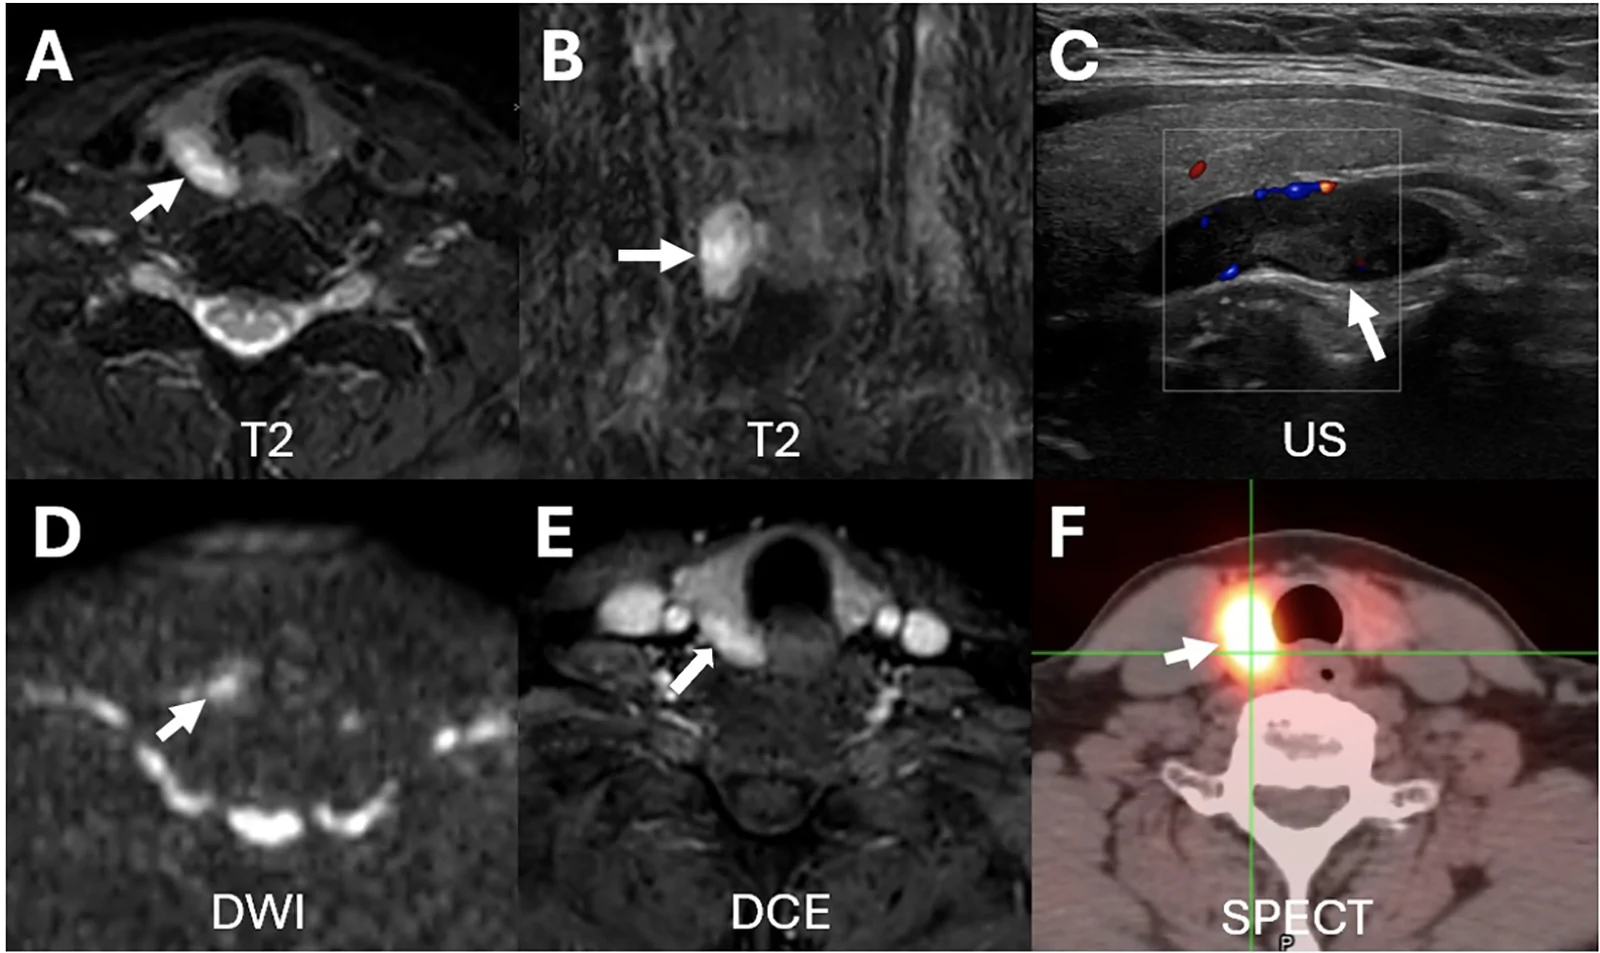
Right upper parathyroid adenoma on MRI in T2 weighted DIXON (A, B), diffusion weighted imaging (D), dynamic contrast enhancement (E), on ultrasound [US, (C)], and single-photon emission tomography SPECT/CT, (F)].
